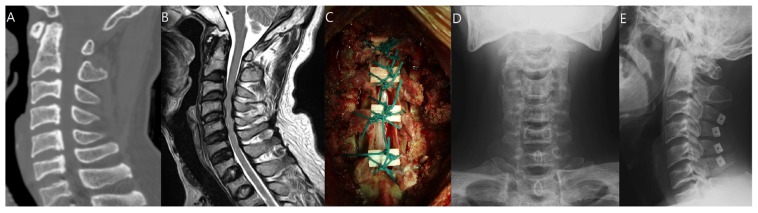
Pre-operative CT (A), pre-operative T2-weighted MRI (B), intra-operative photo (C), and post-operative AP (D) and lateral X-ray (E) of a 49-year-old male patient who underwent C3-6 midline splitting double-door type (French door type) laminoplasty.
